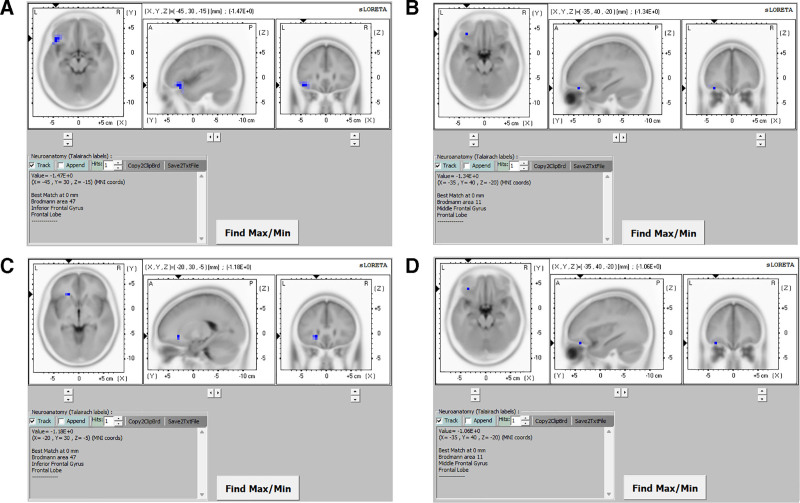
Statistical maps of the 4 EEG frequency bands (delta, theta, alpha, and beta) generated using sLORETA were projected onto a brain MRI template to compare pre- and posttreatment conditions. SnPM analyses were conducted to assess differences in current density distributions between the 2 conditions. The regions showing the greatest current density differences were (A) left inferior frontal gyrus for the delta band, (B) left middle frontal gyrus for the theta band, (C) left inferior frontal gyrus for the alpha band, and (D) left middle frontal gyrus for the beta band. A = anterior, EEG = electroencephalography, L = left, MNI coord = Montreal Neurological Institute coordinate, MRI = magnetic resonance imaging, P = posterior, R = right, sLORETA = standardized low-resolution brain electromagnetic tomography, SnPM = statistical nonparametric mapping.
